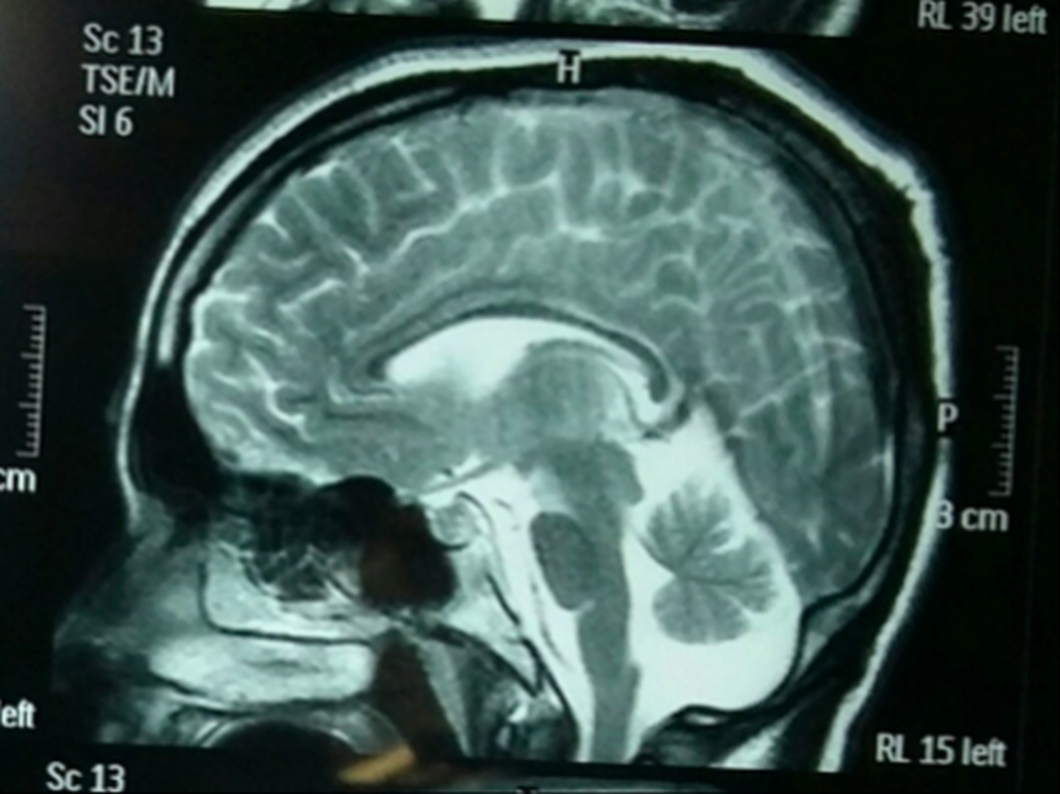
MRI Revealing Cerebellar Atrophy with Dandy-Walker Variant
